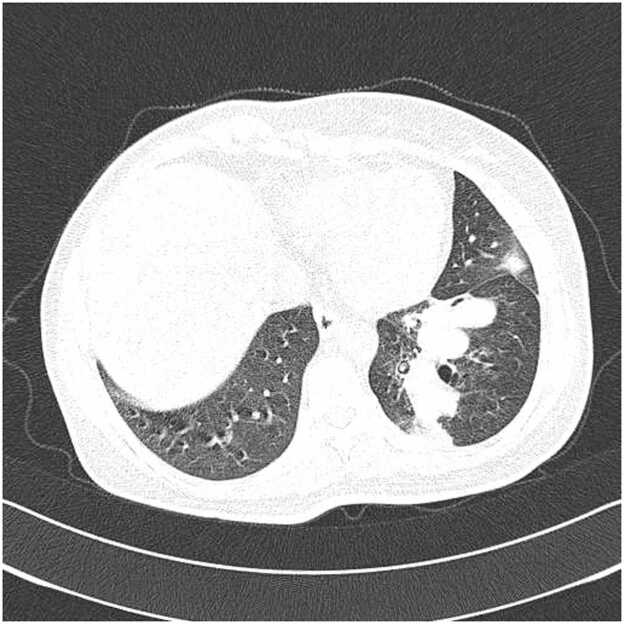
Follow-up HRCT after one month of voriconazole therapy showing treatment response. A. Serial axial HRCT images demonstrating significant improvement with marked reduction in the left lower lobe consolidation. The previously noted cavitary lesion has largely resolved, with only residual thin-walled cystic changes and minimal scarring remaining. The surrounding ground-glass opacities have cleared, indicating resolution of the inflammatory process. These findings confirm excellent response to antifungal therapy. B. Single axial HRCT image at approximately the same level as Fig. 2B, demonstrating significant treatment response after one month of voriconazole therapy. The parenchymal consolidation in the left lower lobe has markedly decreased in size and appears more organized. The previous cavitation has evolved into smaller, more regular cystic or cleft-like changes. The surrounding ground-glass opacity has substantially diminished, indicating resolution of active inflammation. These findings represent excellent therapeutic response to antifungal treatment.
